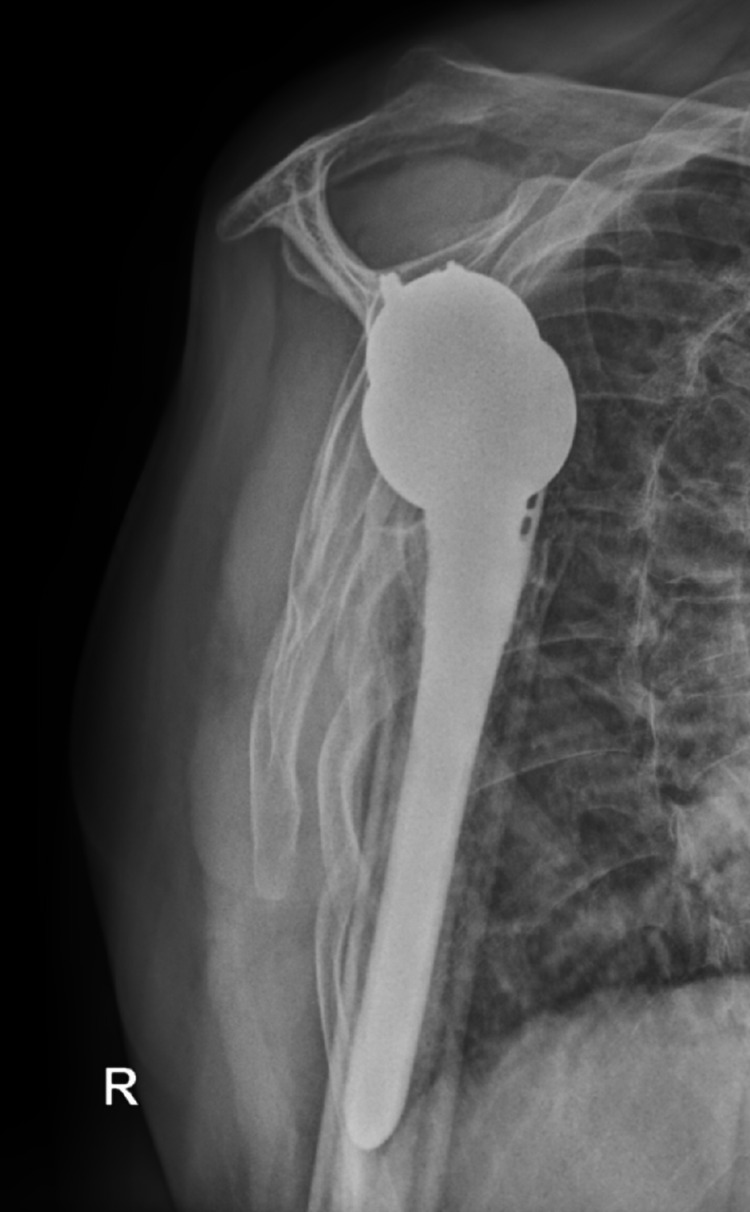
Scapular Y postoperative radiograph of the right shoulder at 12 weeks demonstrates a reverse total shoulder arthroplasty (rTSA) in satisfactory alignment without signs of loosening or failure.
